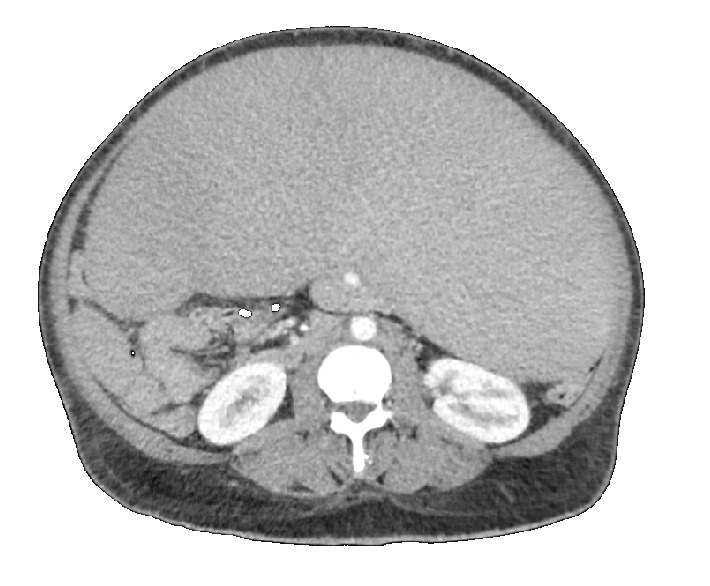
Preoperative computed tomography scan demonstrating marked splenomegaly.
